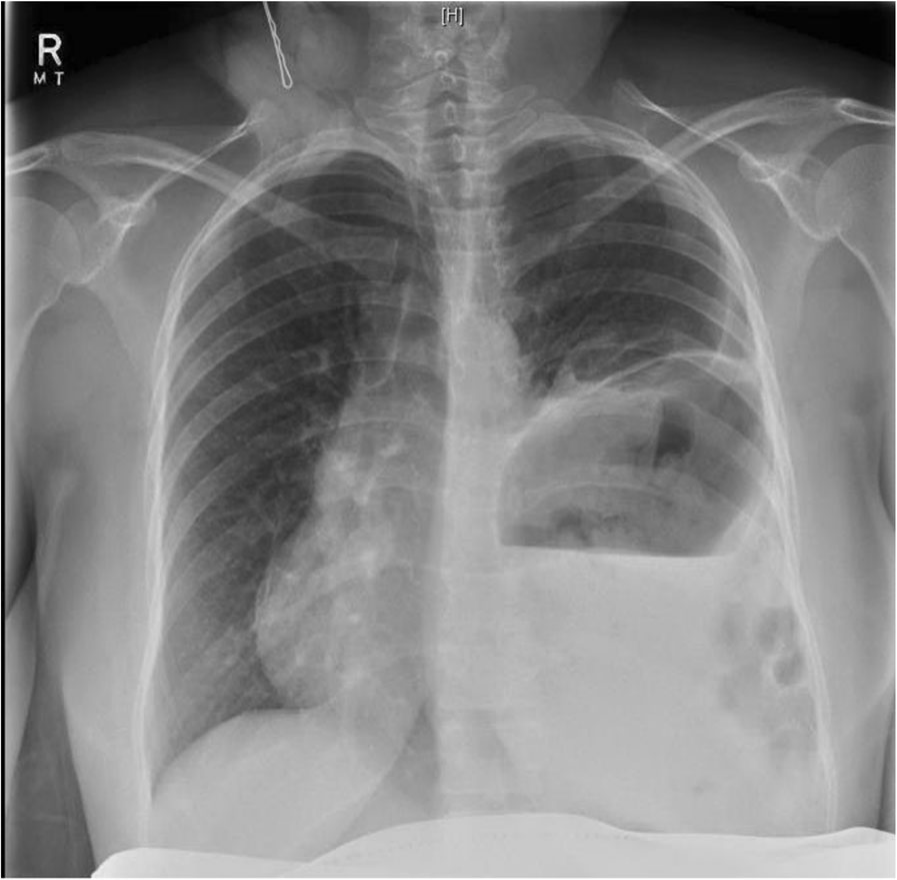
Chest Xray from presentation at 31 + 3 weeks showing a raised or ruptured left hemi-diaphragm with bowel visible in chest and displacement of the mediastinum to the right
